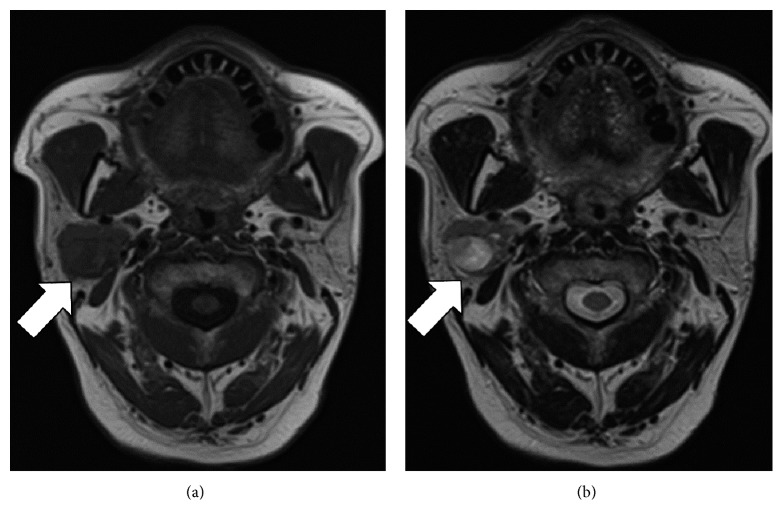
MR imaging findings. (a) A T1-weighted image showing the tumor to have lower intensity than that of the native parotid tissue. (b) A T2-weighted image showing the tumor to have an intermediate signal intensity and partial hyperintensity.
